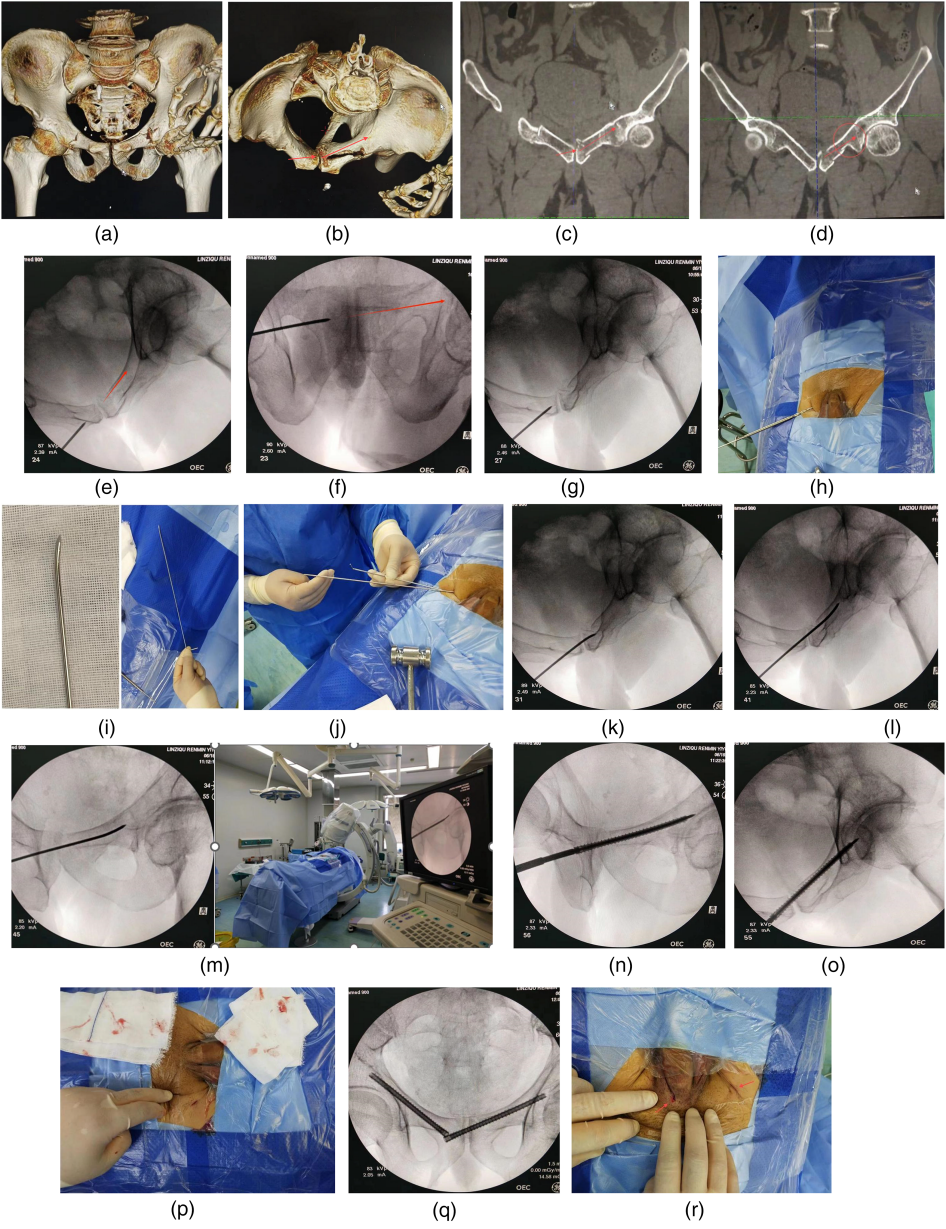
(a) Pelvic fractures included both pubic branches and left sacral wing. (b) The left superior pubic branch split laterally and involves the symphysis pubis. The fixation position was designed and labeled by the arrow. (c) The feasibility of transpubic symphysis screw fixation was confirmed and the puncture path was planned by coronal CT scan. (d) It was clear that there was a relatively intact anchoring area at the acetabular fracture end, which was largely surrounded by three sides of cortical bone. (e) Pelvic inlet fluoroscopy: The extension line of the guide pin was close to the posterior cortex of the pubic branch on the affected side. (f) Pelvic outlet fluoroscopy: The extension line of the guide pin was located between the upper margin of the obturator foramen and the central axis of the pubis designed for this patient. (g) Hammered the guide pin into the bone about 1 cm. (h) Used a hollow drill to enlarge the bone inlet. (i) Took another guide pin of the same size and shaped it for use. (j) Replaced the guide pin after exiting the hollow drill. (k) Both sides of the pubic symphysis were penetrated with shaped guide pin. (l) Adjusted the direction of the guide pin by adjusting the direction of the guide pin end to follow the posterior margin of the pubic branch. (m) Adjusted the perspective direction to ensure that the path was long enough without interfering with the acetabulum. (n) A hollow screw of 6.5 mm diameter was nailed along the guide pin. (o) The fracture alignment was well observed by pelvic inlet fluoroscopy. (p) Incision size, appearance and total blood loss (gauze impregnation). (q) The contralateral pubic rami were subsequently fixed with retrograde hollow nail. (r) The incision appearance of both screws with one of the incisions was already closed.
